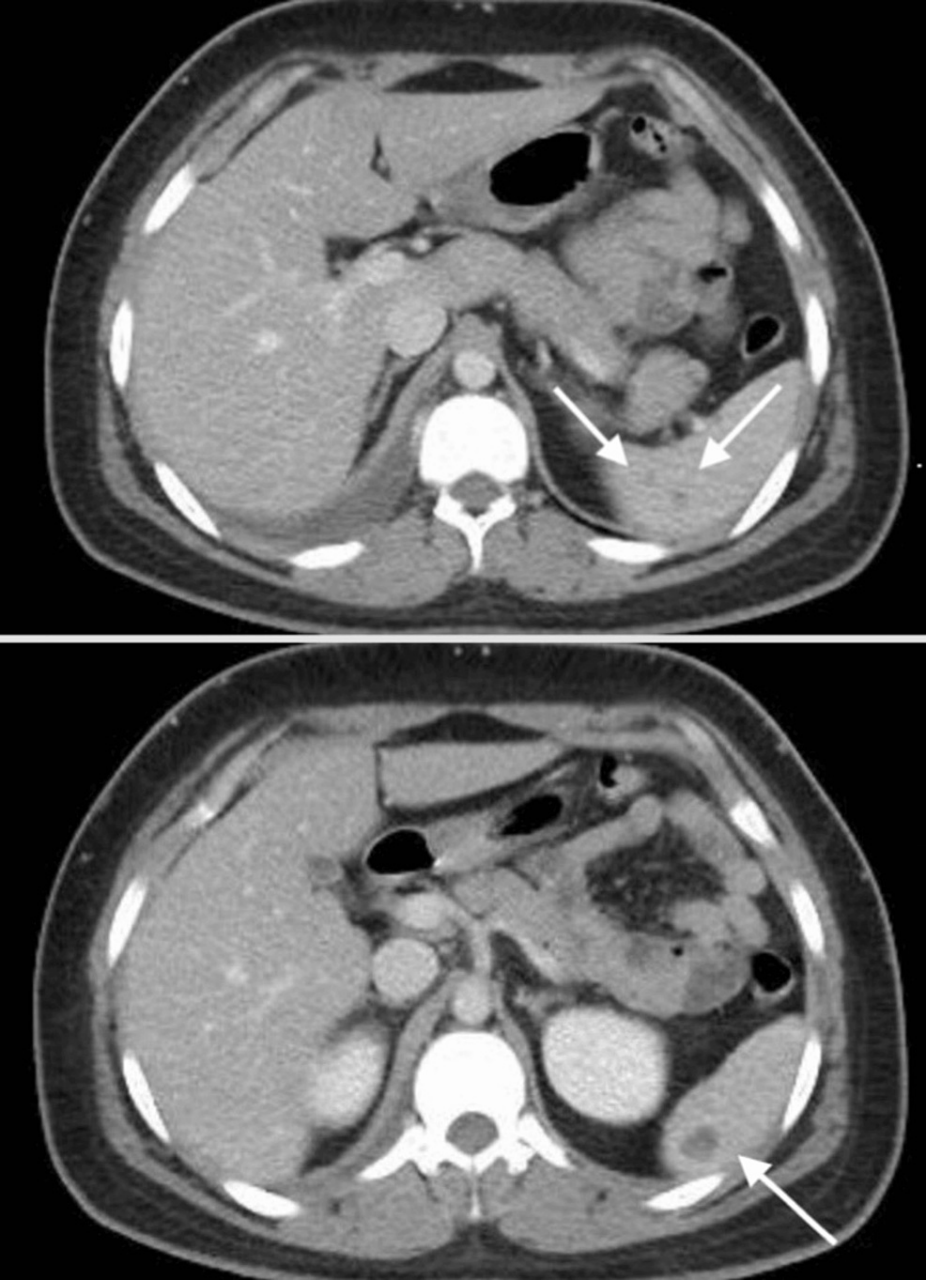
Computed tomography scan of the abdomen. Axial CT scan of the abdomen showing two splenic lesions (white arrows).
